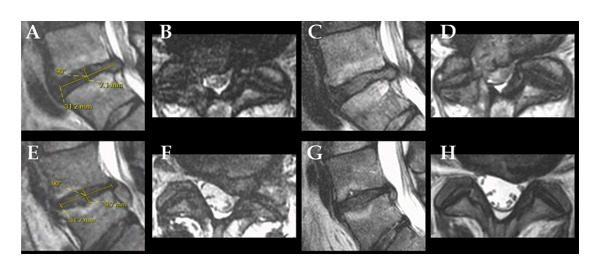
Demonstration of disk measurements. Example of patient without clinical improvement without any intervention with extrusion and middle disc height below the threshold (upper row) in sagittal plane (A, C) and axial plane (B, D) of the first MRI examination (A, B) and the second (C, D). Example of patient with clinical improvement without any intervention with extrusion and middle disc height above the threshold (lower row) in sagittal plane (E, G) and axial plane (F, H) of the first MRI examination (E, F) and the second (G, H).
